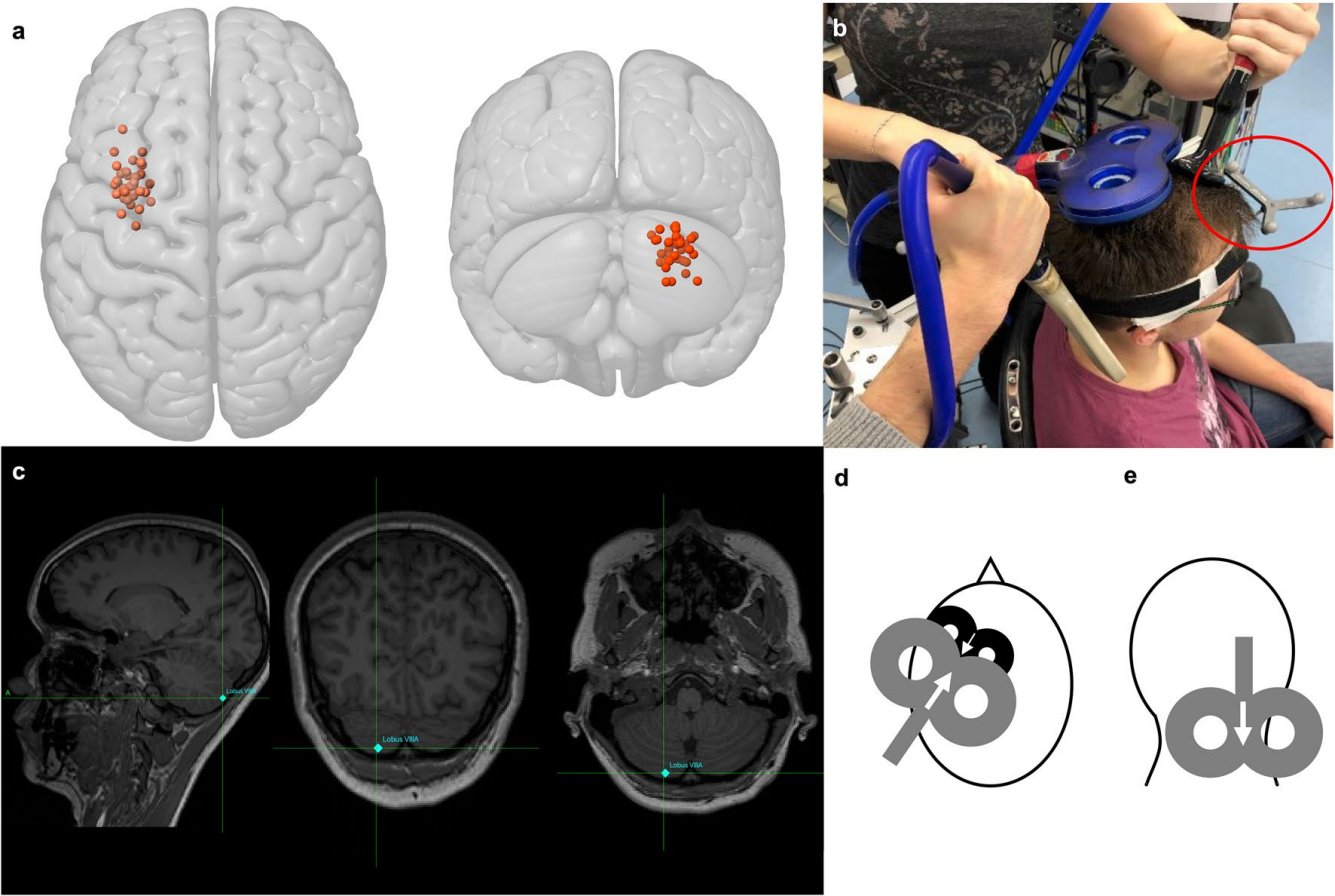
Coil positions and stimulation sites. (a) Individual stimulation sites for right lobule VIIIA (red) and dorsal premotor cortex (PMd) (orange) using the Montreal Neurological Institute and Hospital (MNI) stimulation coordinates in anatomical convention. (b) Neuronavigated triple-coil transcranial magnetic stimulation (TMS) experimental setup. The red circle marks the position of the tracer with three reflecting spheres on the subject’s head (staff member, that gave permission to publish the picture). TMS coils are located over PMd (small 25 mm black coil) and cerebellum stimulation site (grey figure of eight coil). Blue 70 mm coil is located over the primary motor cortex (M1) hot spot. (c) Magnetic resonance T1-weighted image (MRI) of one participant with targeted stimulation spot in the right lobule VIIIA at the cerebellar surface using radiological convention. (d) View from above. The black coil symbolizes the 25 mm branding-iron-coil positioned over the left PMd. White arrow indicates TMS current flow in anterior-to-posterior direction. Grey coil symbolized 70 mm coil positioned over M1 and slightly overlapping with PMd coil. White arrow indicates TMS current flow in posterior-to-anterior direction. (e) View from behind. The grey coil symbolizes the 70 mm coil positioned over the cerebellum (lobus VIIIA) with handle pointing cranially.
